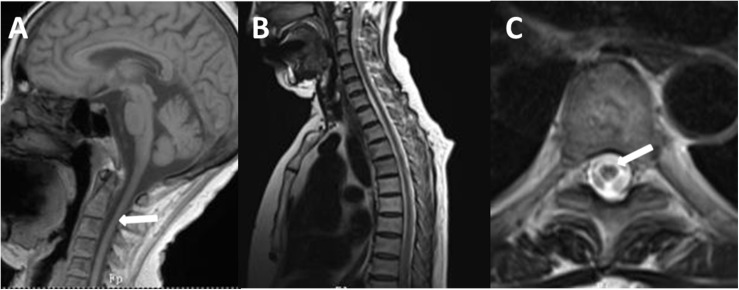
T1-weighted spin-echo image (T1W) (A), T2W (B), and cross-sectional (C) MR image of the spinal cord of the proband. T1W on sagittal surface and cross-sectional MRI showed diffuse withering, with accompanying abnormal signal in white matter of thoracic one to four level spinal cord on T2WI.
